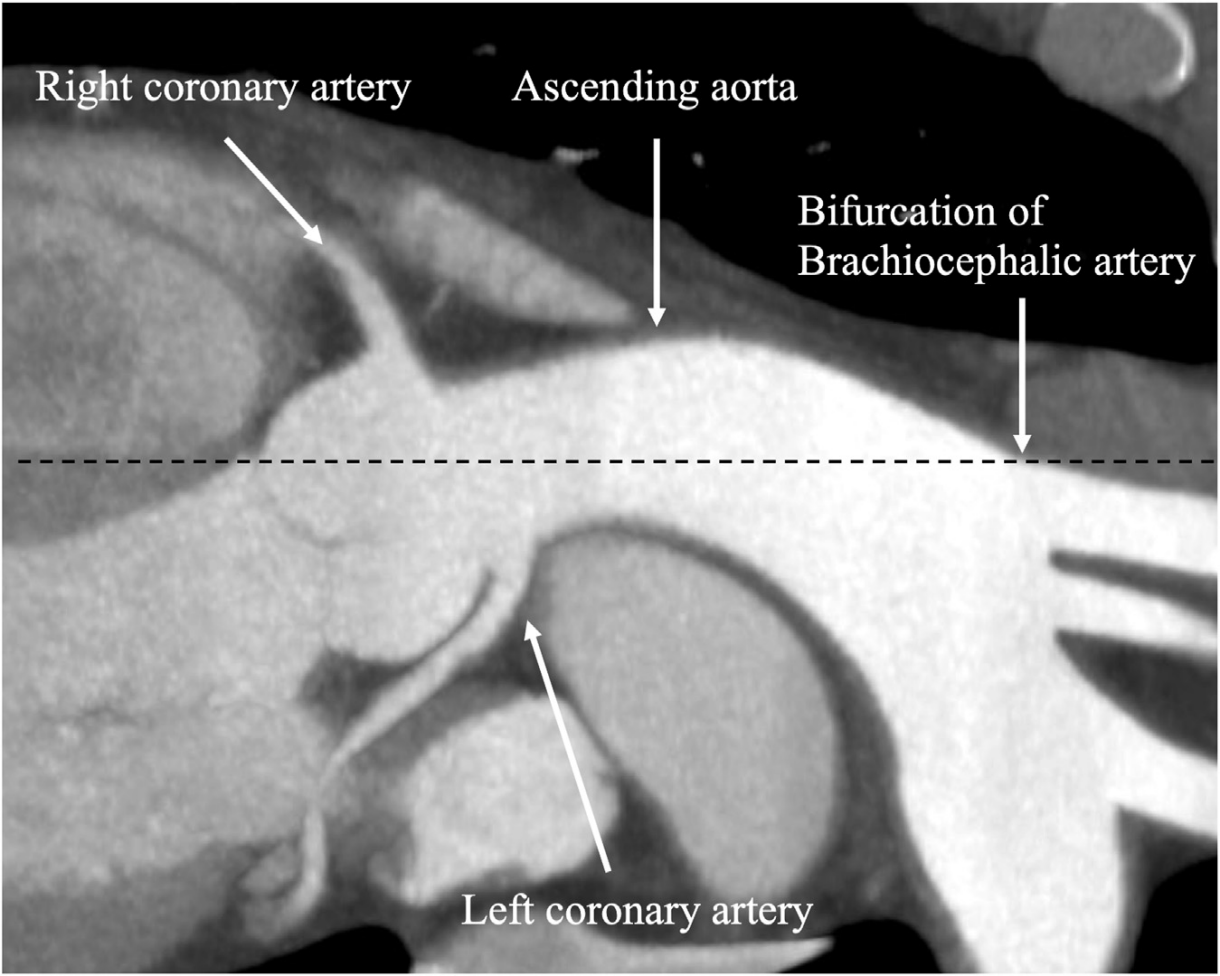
Positional relationship between aortic vessels and branches. Contrast computed tomography shows that the anterior wall of the ascending aorta was located higher than the brachiocephalic bifurcation in the supine position. The dotted line represents the height of the bifurcation of the brachiocephalic artery.
